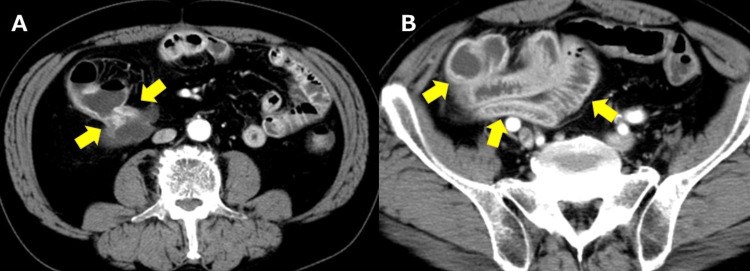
Contrast-enhanced abdominal CT. (A, B) Axial images show a tight ileocolic anastomotic stricture with upstream small-bowel dilatation and mural hyperenhancement; low-attenuation tissue contiguous with the anastomosis (arrows) suggests an extramural fatty component.
